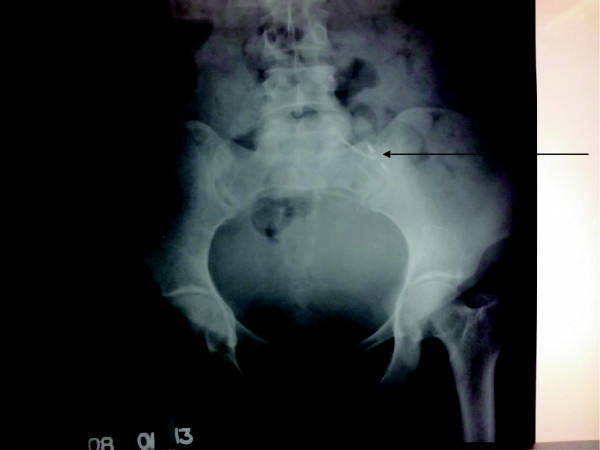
Anteroposterior X-ray of pelvis showing the intrauterine device (arrowed).
